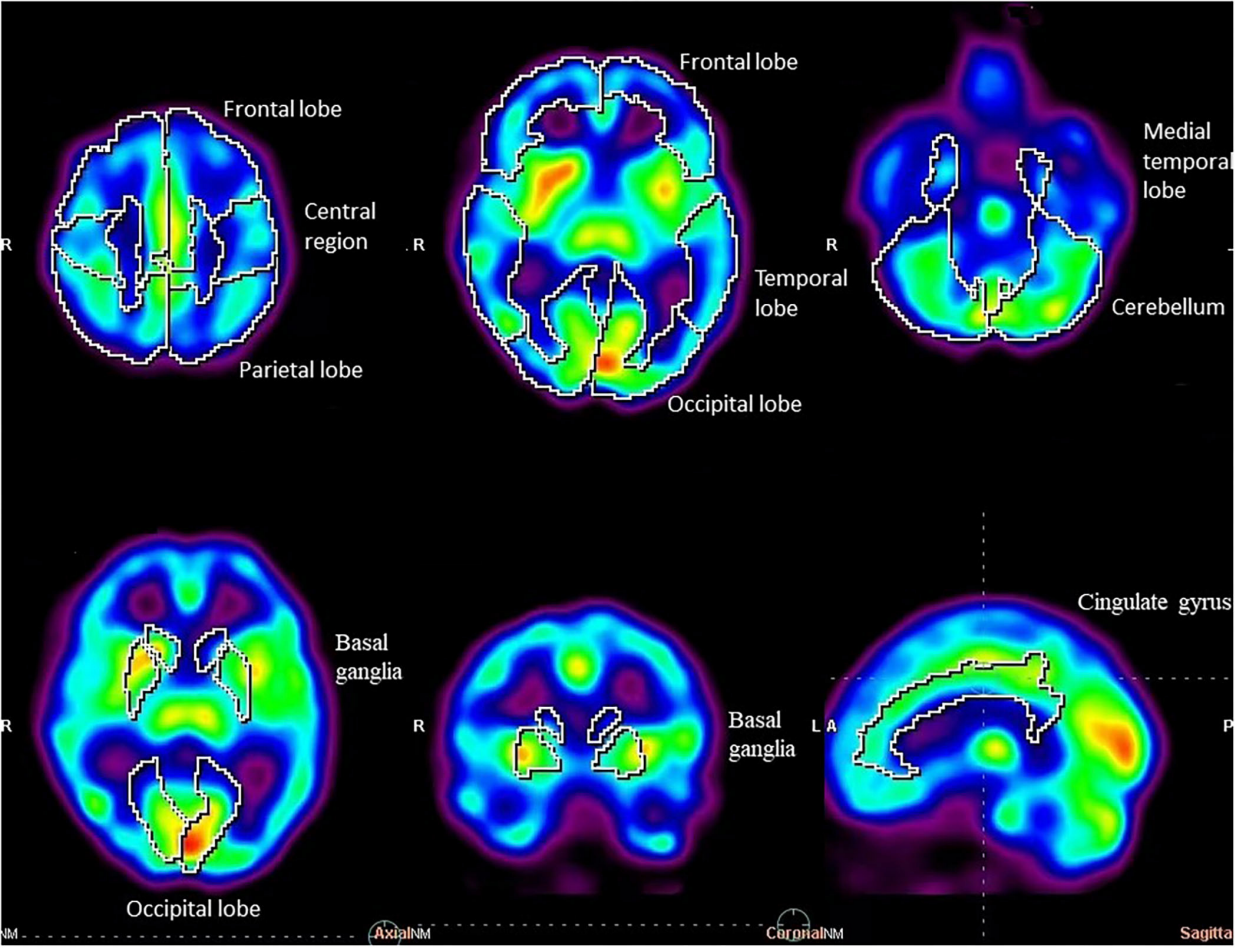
SPECT standard regions of case 15, a 23-year-old woman.
